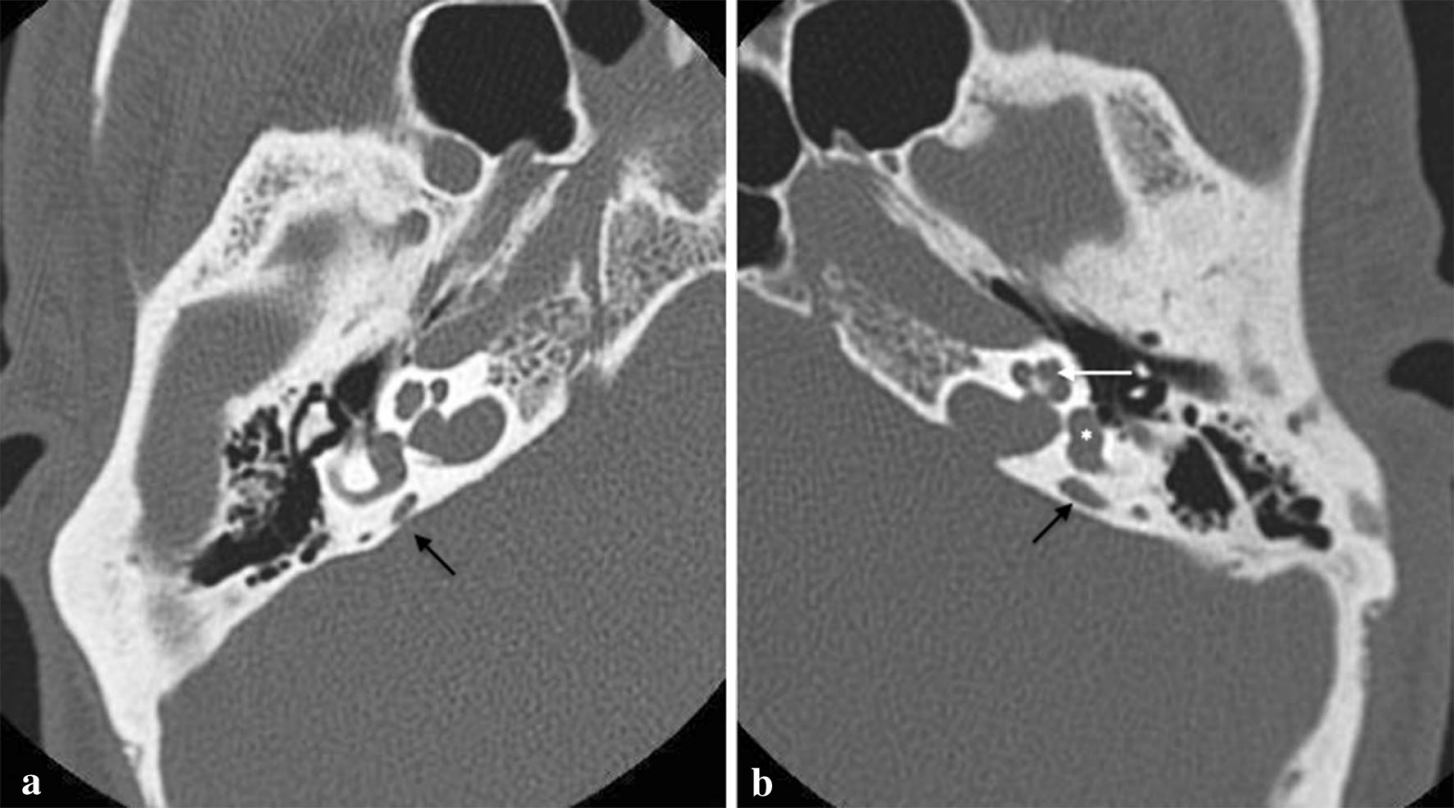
Enlarged vestibular aqueduct (EVA). A 7-year-old female with progressive bilateral SNHL. Axial CT obtained at IAC level show bilateral EVA syndrome. The cochlea (white arrow in b), the vestibule (asterisk in b) and SCCs have a normal appearance but there is a large vestibular aqueduct (black arrows in a and b)
